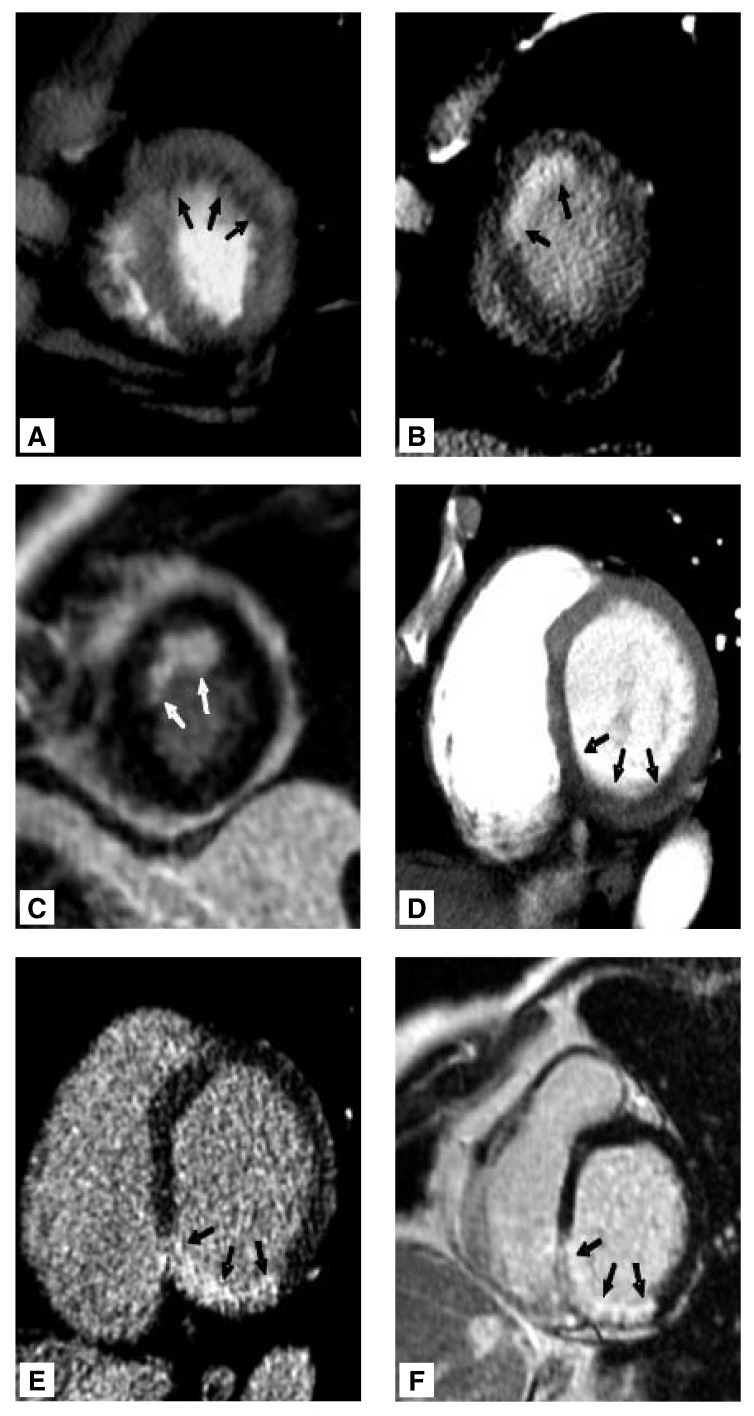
Arterial-phase CT depicts myocardial infarction as area of reduced contrast enhancement (A, D), while late-phase CT shows a delayed enhancement in the area of infraction (B, E) (arrows). CT can differentiate subendocardial (A−C) from transmural infarction (D−E). Extent and location of actual infarction on delayed enhanced CT (B, E) correlate better with MR imaging (C, F) than arterial-phase CT (A, D).
